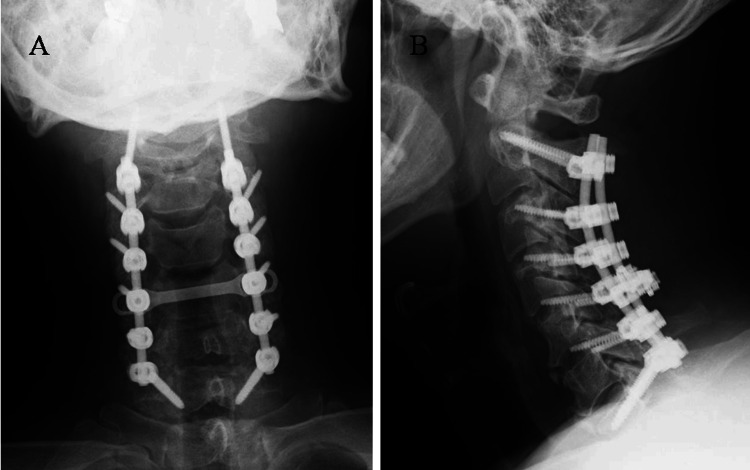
X-ray after cervical decompression and fusion surgery (A) Frontal view; (B) Lateral view. C3-C5 laminectomy, C2-C7 posterior fusion, and dome-like laminectomy of C2 were performed.
